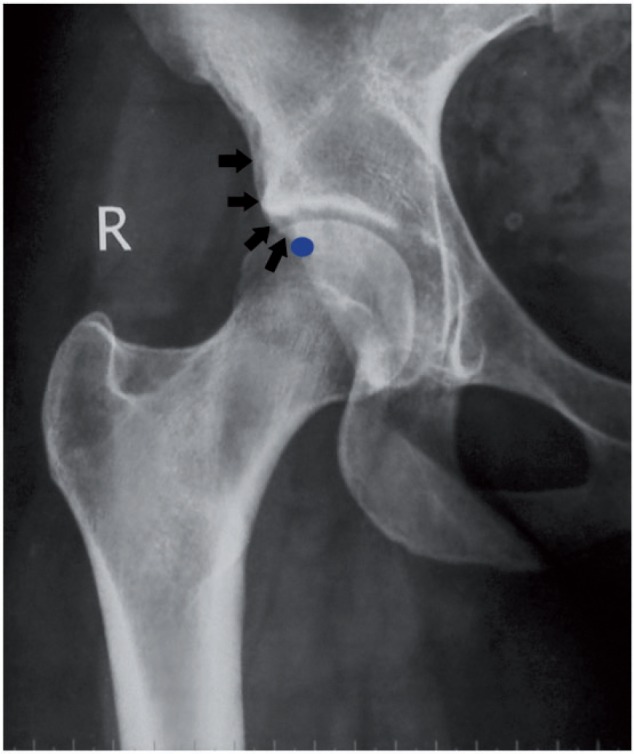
Close-up view of the right hip from an anteroposterior plain radiograph of a 19-year-old female with positive crossover sign. The point of crossover is marked by a blue dot. Arguably, the AIIS continues distally with round borders (black arrows) extending below the acetabular margin, potentially contributing to a false appearance of acetabular retroversion. Such a patient would require further investigation with computed tomography, to include 3-D imaging.
